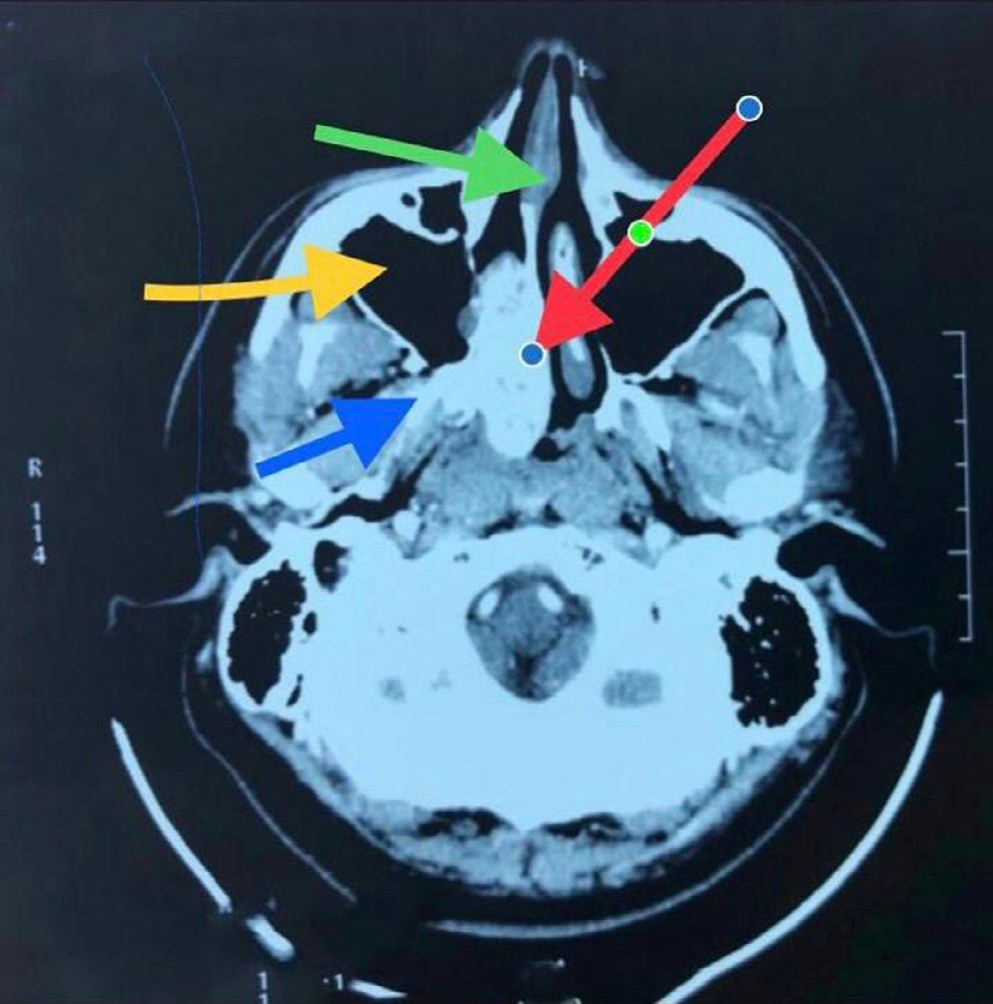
Axial cut of CT findings. Green Arrow: nasal septum. Red arrow: tumor in the nasal fossa and nasopharynx. Yellow arrow: right maxillary sinus. Blue arrow: tumor extension to the pterygopalatine fossa
